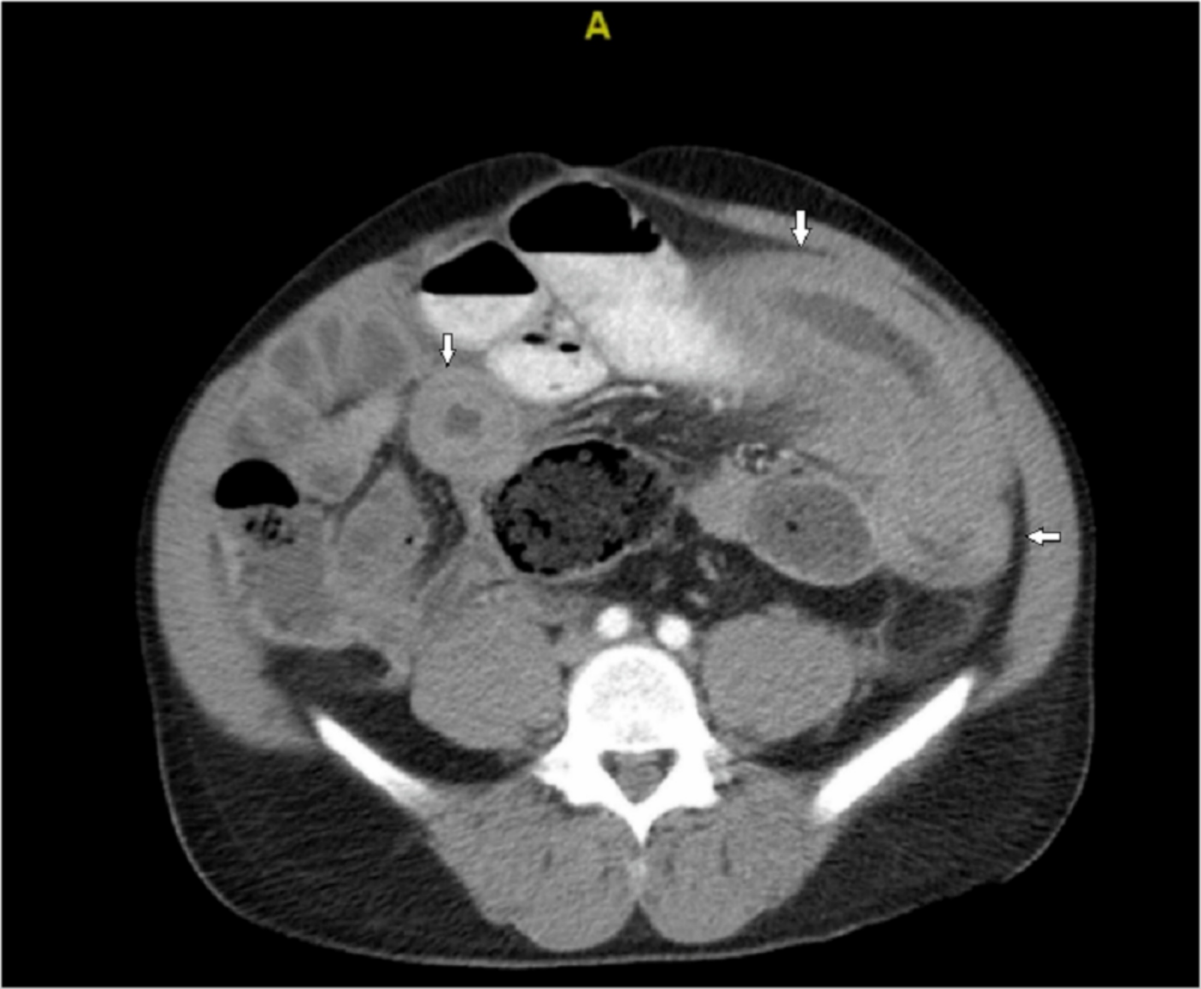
A CT scan of the abdomen revealing thickened small bowel
